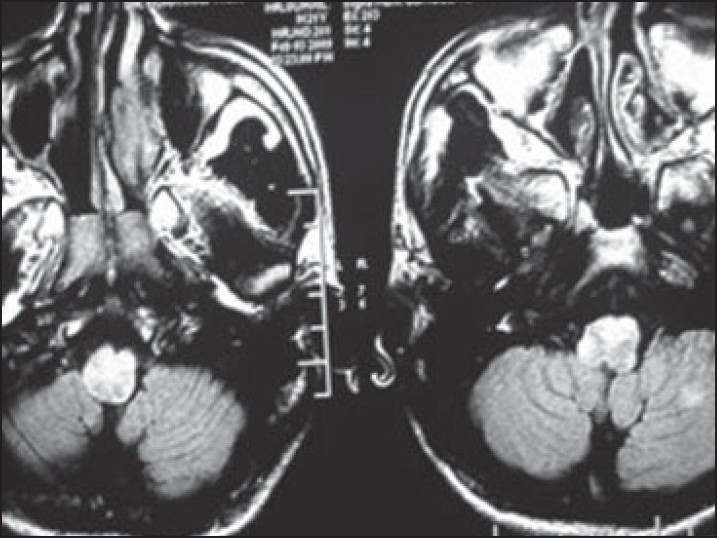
The medullary region showing a hyperintense signal on FLAIR, an atypical and rare distribution of PRES.
